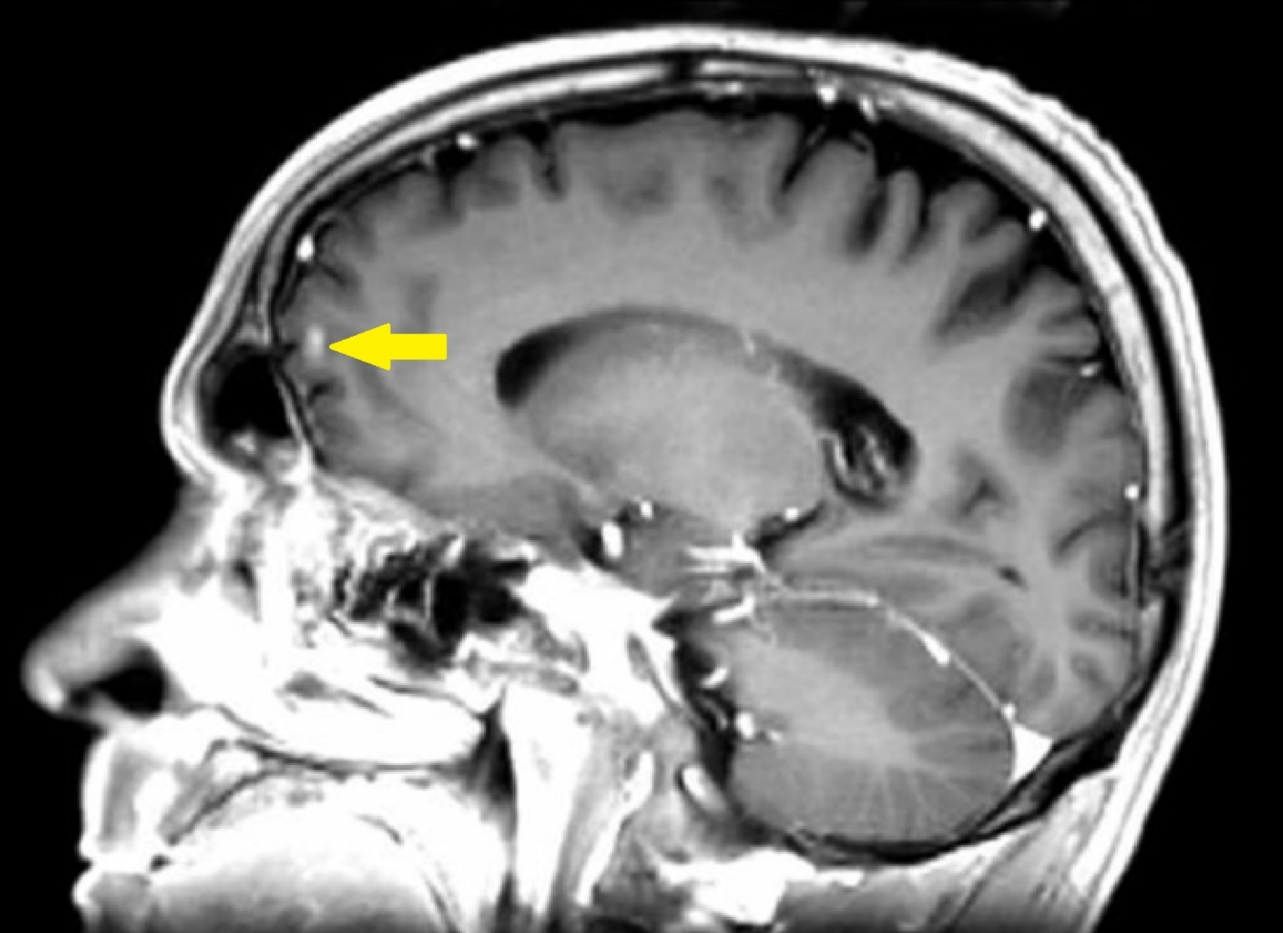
Left Frontal Lobe Metastasis Reduction Post-Treatment Sagittal Brain Magnetic Resonance Imaging (MRI).
